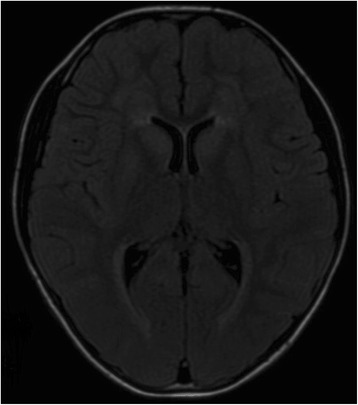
Axial FLAIR image of the asymptomatic daughter of our patient show band-like hyperintense lesions around the lateral ventricle
